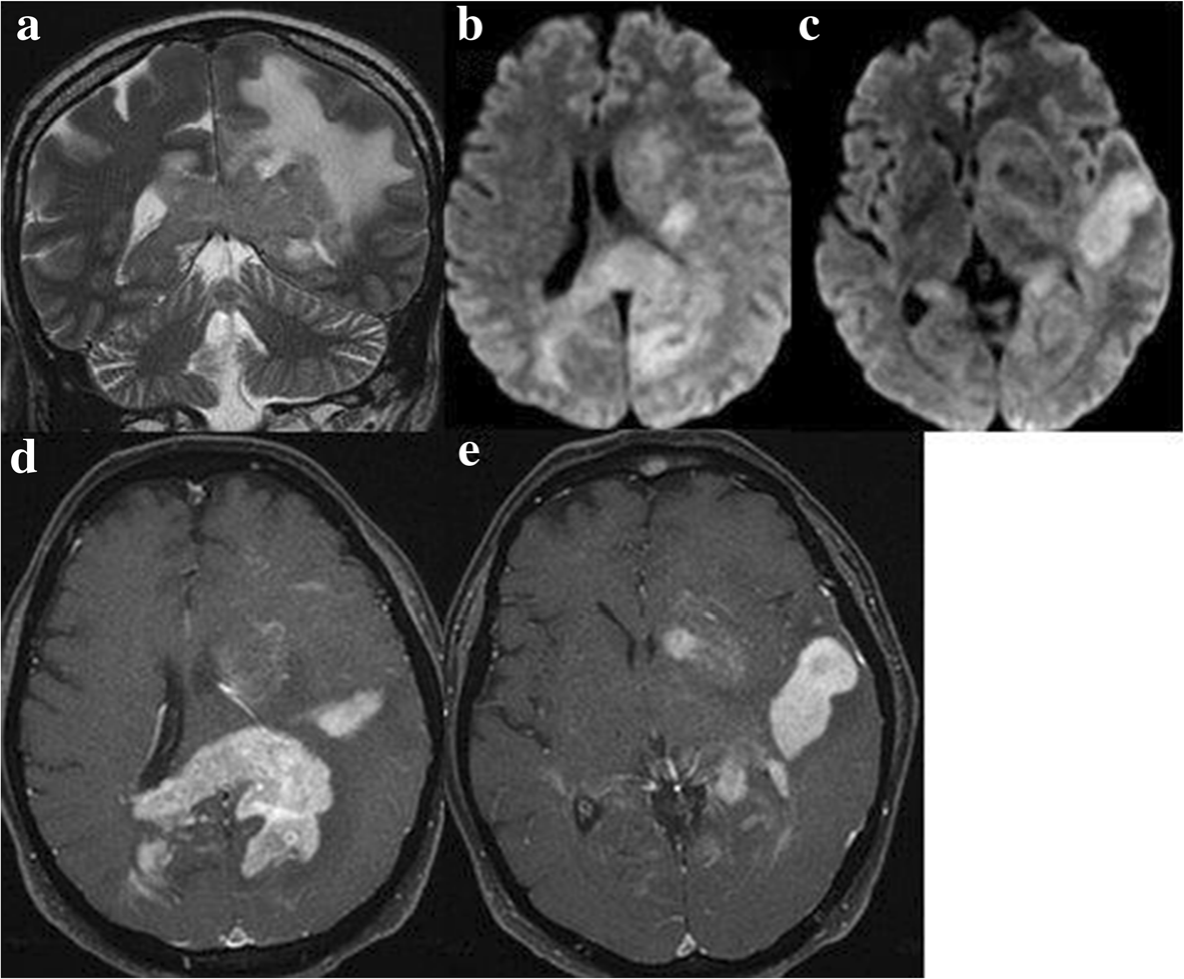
MRI findings strongly suggestive for brain lymphoma: SE T2 sequences in coronal plane (a), diffusion in axial plane (b, c) and fat-suppressed T1 after contrast medium infusion in axial plane (d, e). MRI shows multiple bilateral, periventricula sites with low signal intensity on T2, with oedema, hyperintensity signal on DWI and homogensously enhancement after contrast medium infusion
